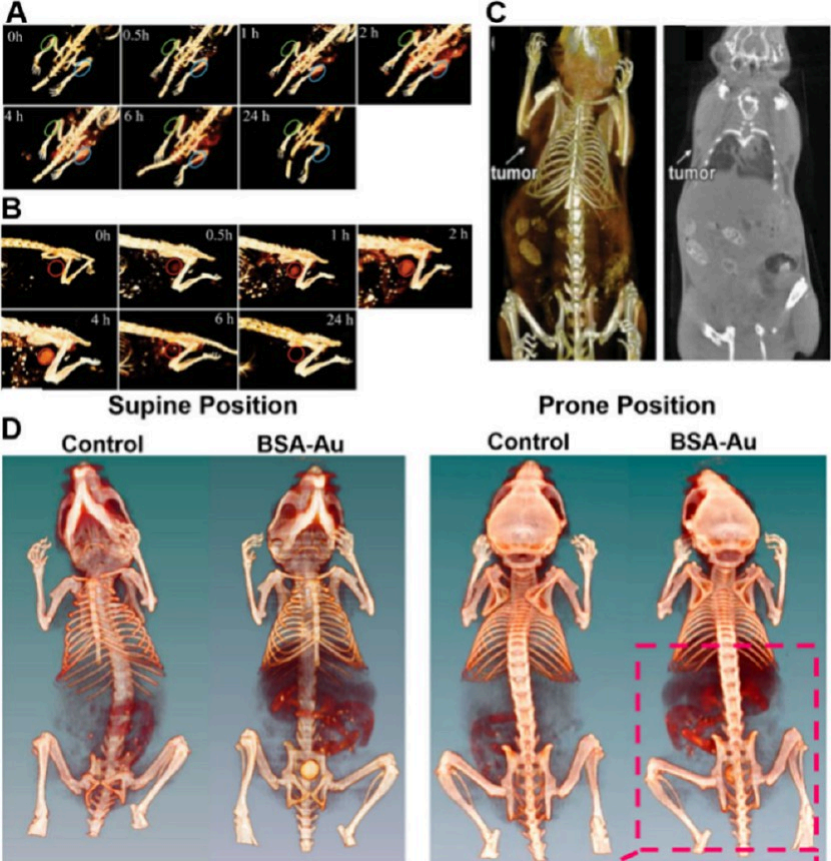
(A) *In vivo* 3D CT images of the PC3pip
